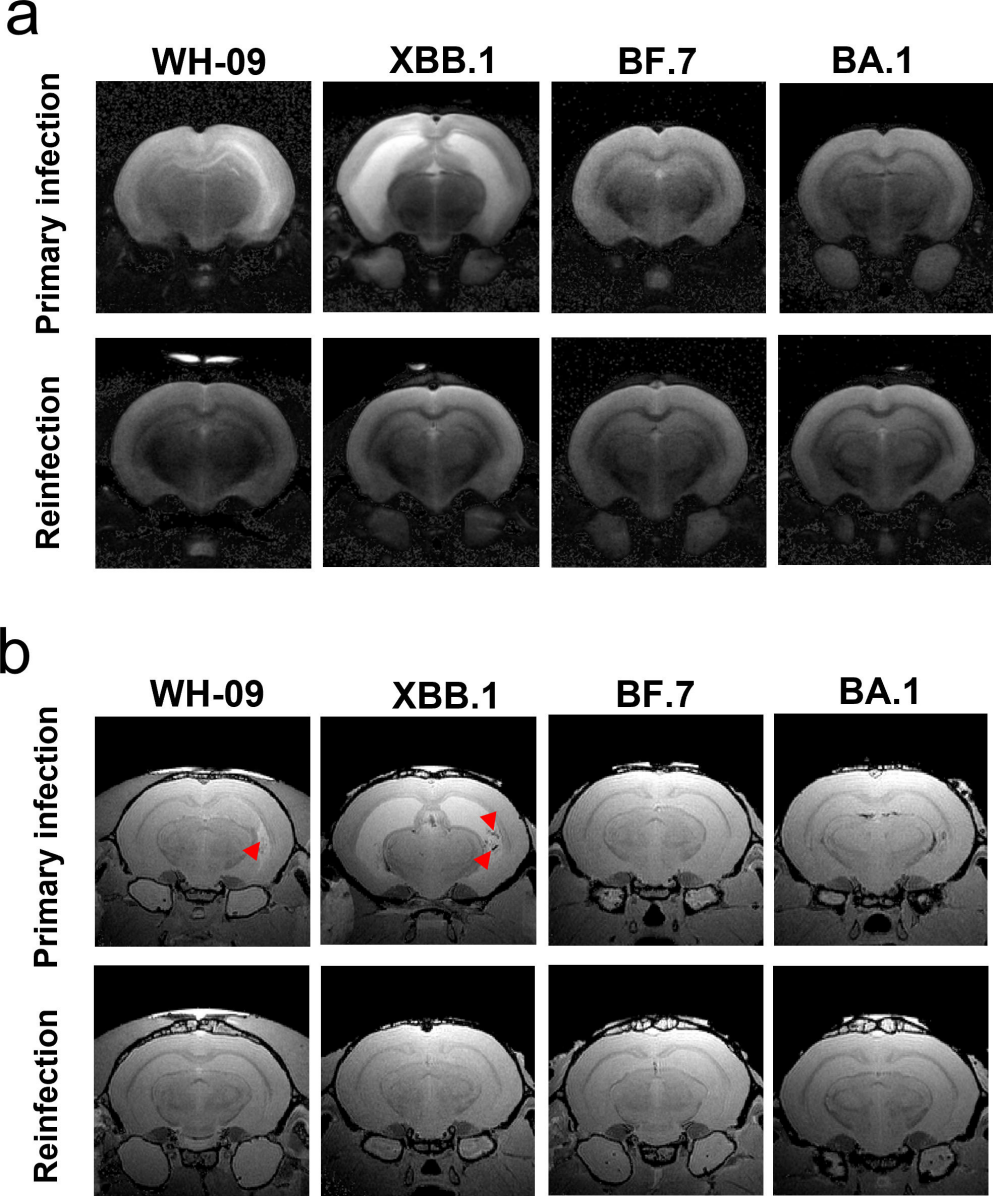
T2 (a) and T2* (b) brain MRI images of a hamster with primary infection and reinfection by different SARS-CoV-2 strains. The red triangles indicate hemorrhagic spots.
